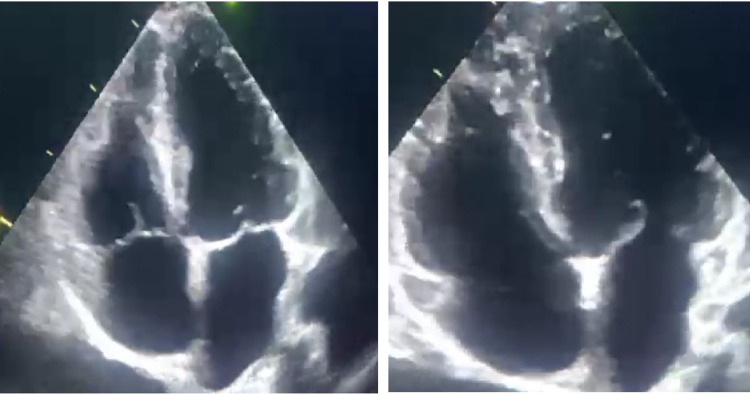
Echocardiography four weeks after anticoagulant initiation Transthoracic echocardiography showing complete resolution of the right ventricular thrombus after four weeks of anticoagulation.
